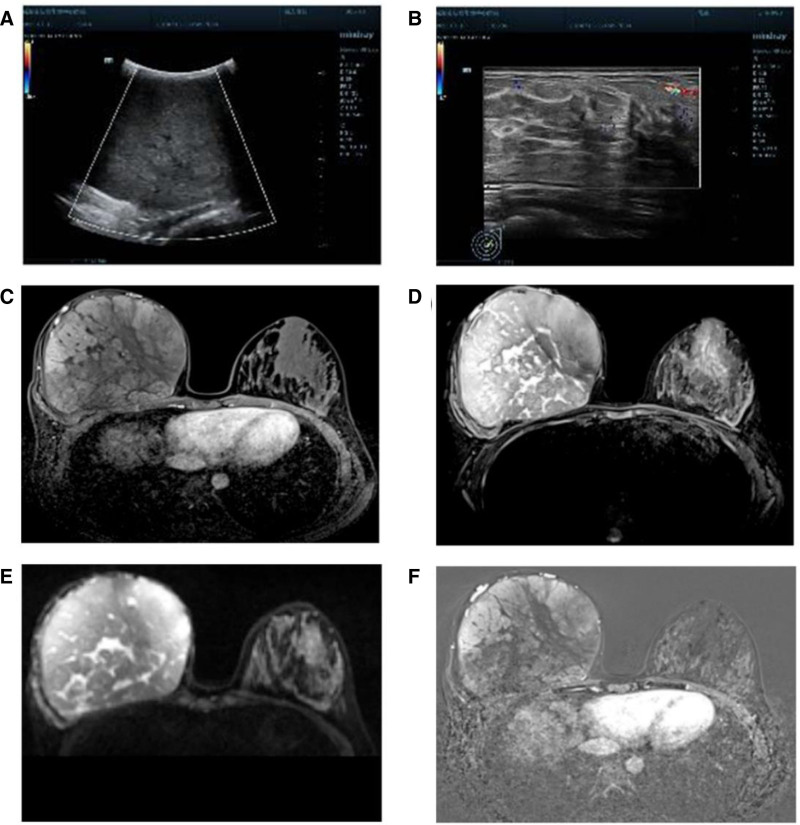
Preoperative ultrasonography (A, B) and MRI (C–F) of bilateral breasts. MRI = magnetic resonance imaging.
